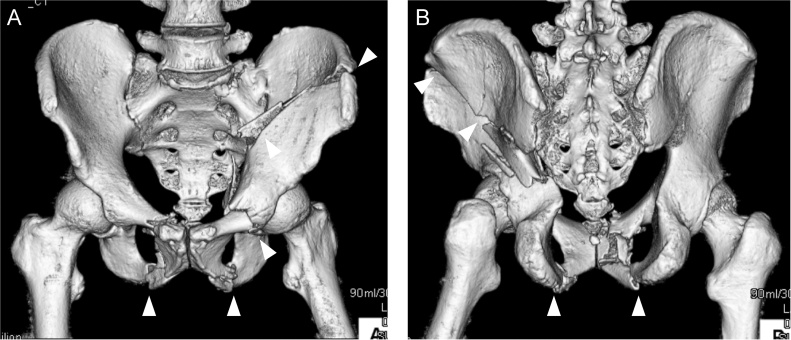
CT image of the pelvis after injury, showing the both-column fracture in the left acetabulum and bilateral pubic bone fractures (arrowheads).
